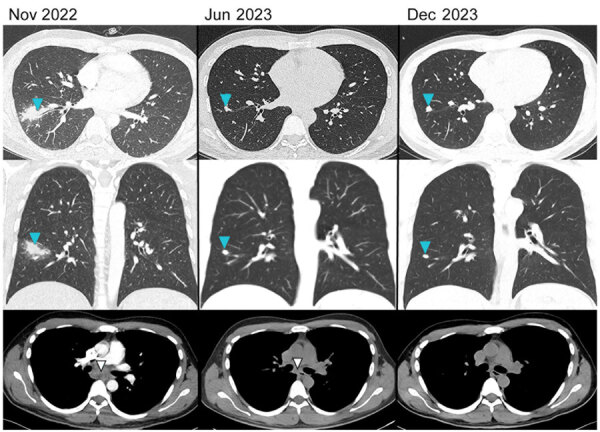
Computed tomography scan findings of a patient with a suspected case of coccidioidomycosis contracted in Peru and the changes in findings seen over 12 months while in treatment in Switzerland, 2022 and 2023. Blue arrows highlight the pulmonary consolidation and regression over time. White arrows highlight the enlarged infracarinal lymphnodes and their regression and normalization over time.
